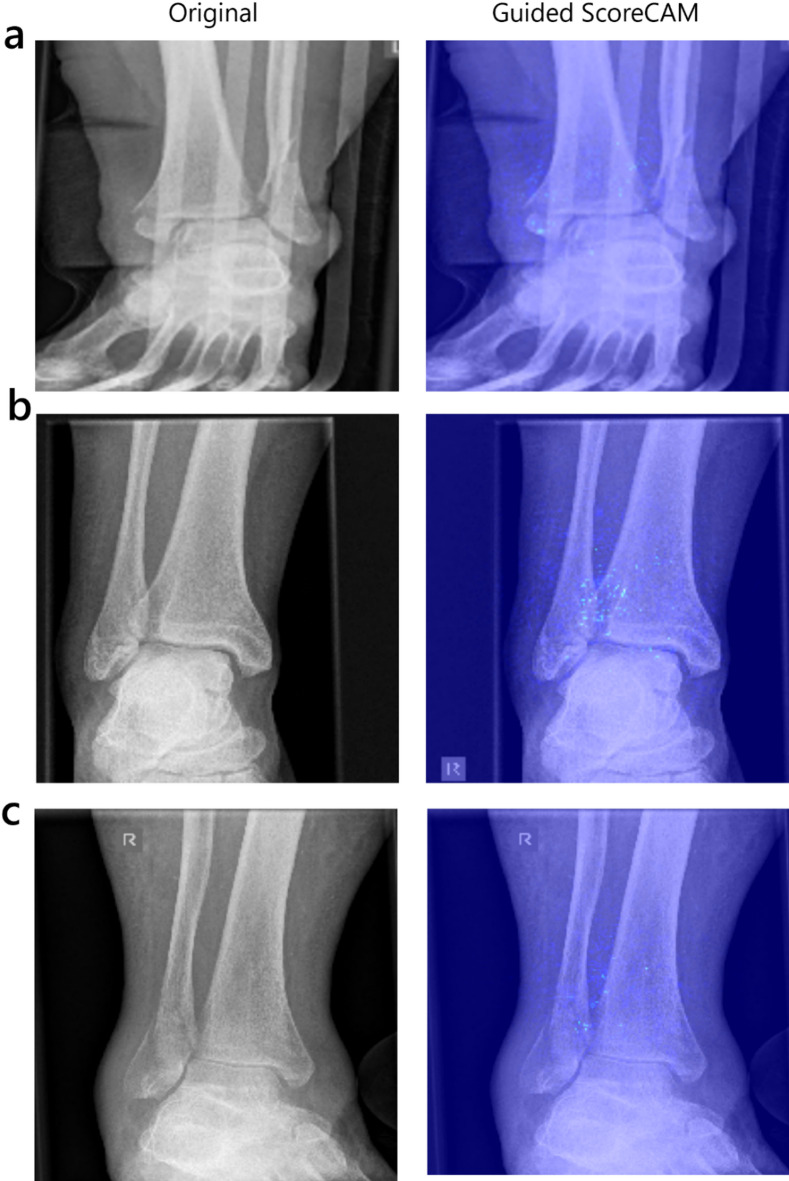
(a): Guided Score-CAM in higher grade fracture dislocation in an AO 44 B2 fracture of a 58 y old female with an unstable syndesmosis. (b) AO 44B1 fracture in a 40 y old female depicting an unstable syndesmosis with minimal radiologic signs in the a.p. x-ray. (c) False positively predicting an AO44 B1 Fracture in a 67 year-old male with Guided Score CAM focusing on the syndesmotic region.
